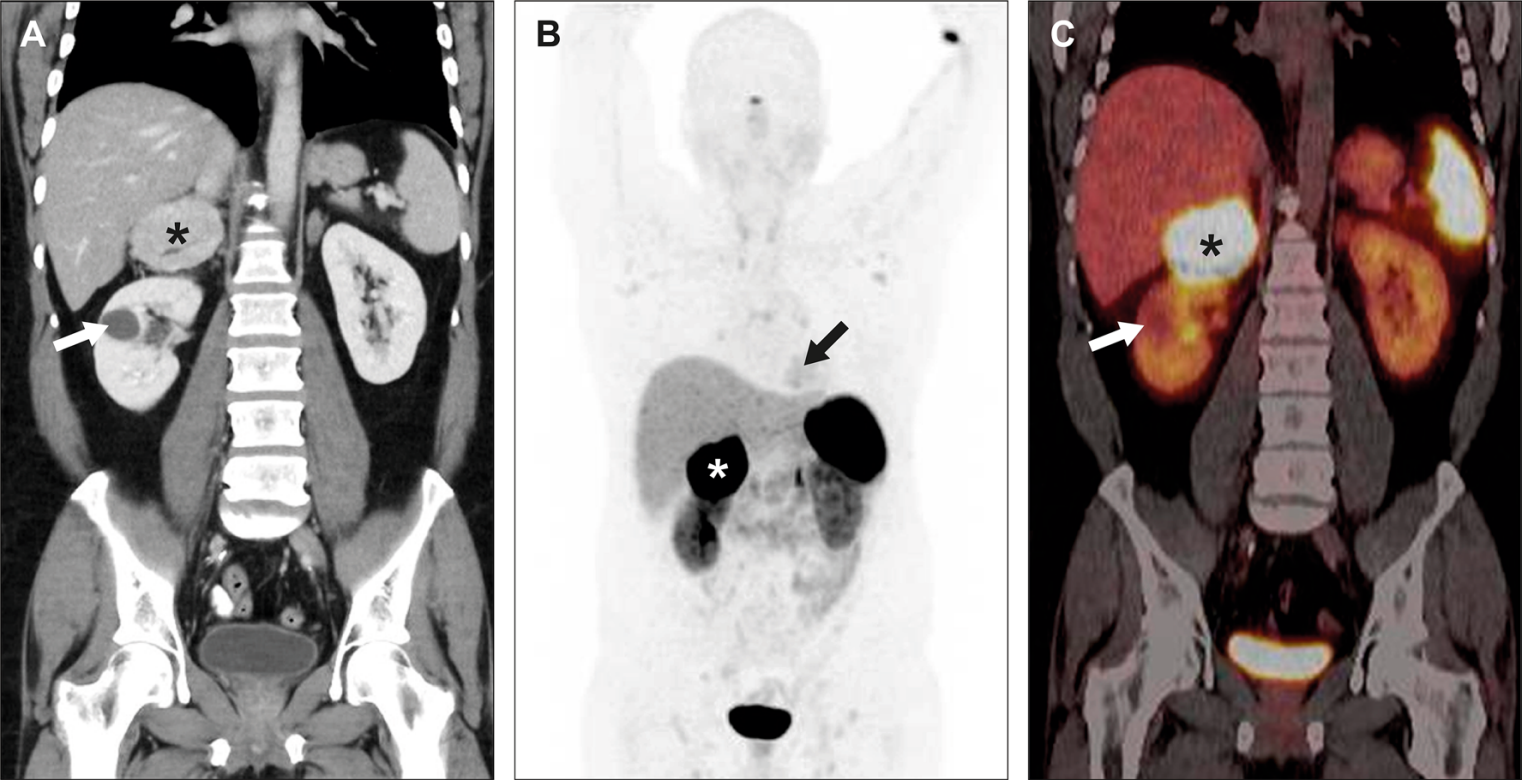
Abdominal computed tomography (A) revealed a large (approximately 7 × 6 × 6 cm) right-sided adrenal mass with central necrosis (as indicated by a black star), which showed clearly pathologic uptake on ^68^Ga-DOTATOC PET/CT (as indicated by a white star in the maximum intensity projection image (B) and a black star in the PET/CT fusion image (C)). Additionally, the maximum intensity projection image (B) displayed a low cardiac uptake (as indicated by a black arrow). Furthermore, a renal cyst was found in the right kidney (as indicated by a white arrow (A and C)). Maximum standardized uptake values: liver 7.5, spleen 35.3, adrenal mass 135.9, and heart 5.0
